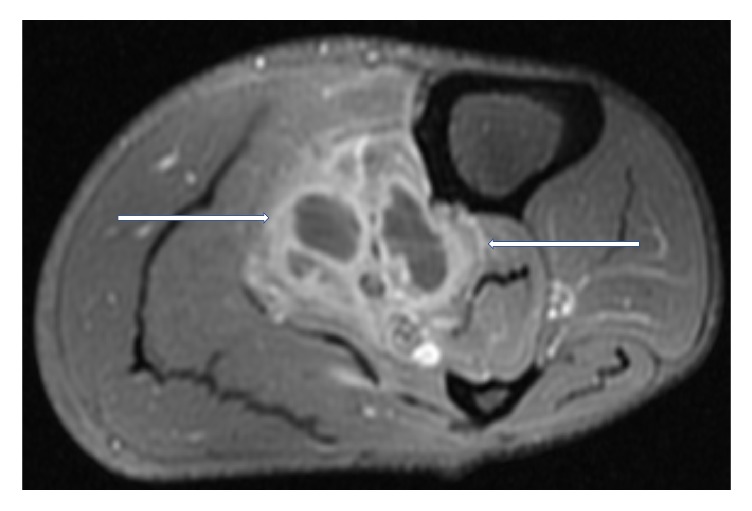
T2 weighted, fat saturated, postcontrast, axial MR image of left calf showing a multiloculated fluid collection with associated enhancing rim and septations (white arrows) within the tibialis posterior muscle, compatible with an intramuscular abscess.
